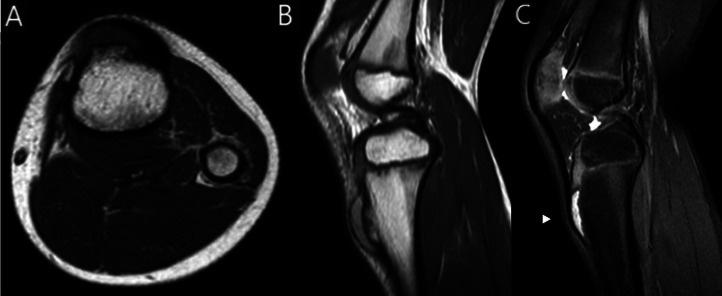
MRI of the left knee. TSE T1w axial (A) and sagittal (B) section showed a protruded bone lesion of the tibial tuberosity with medullary and cortical continuity with the underlying bone. STIR sagittal section (C) better showed the cartilage cup (arrow head) underneath the patellar tendon.
